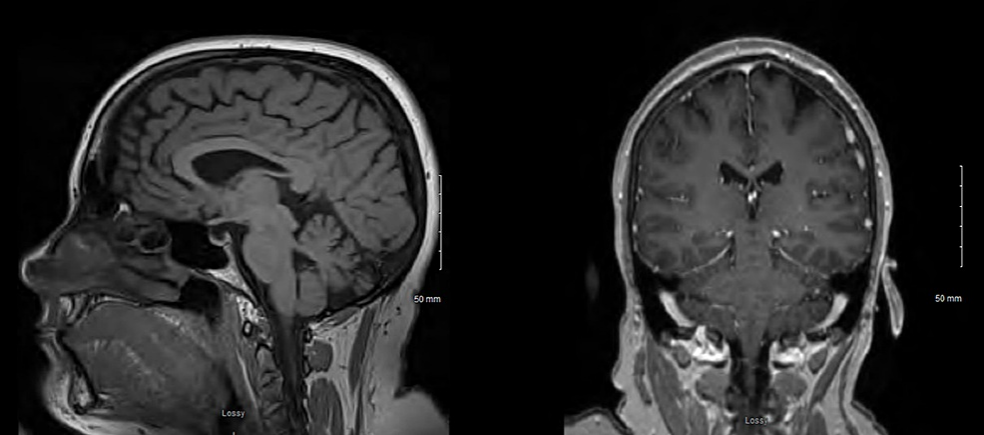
MRI brain results from initial emergency department visit demonstrating small punctate foci inconsistent with multiple sclerosis or acute neurologic sequelae and no evidence of cervical spine demyelination
